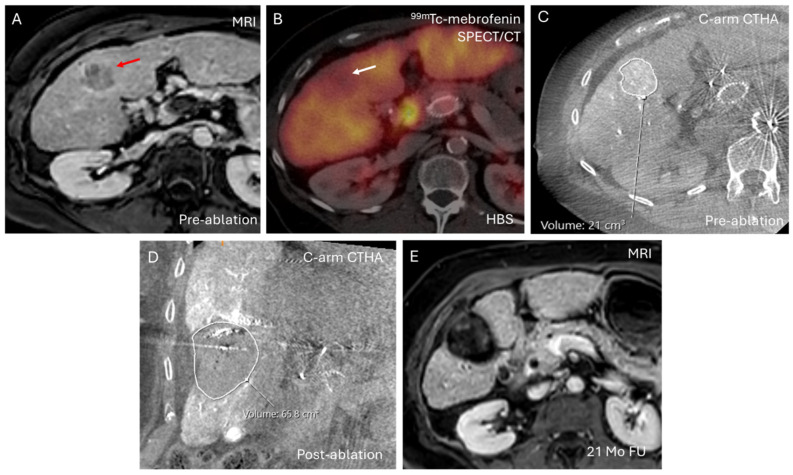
Case example of a patient with severely impaired liver function (1.6%/min/m^2^) who underwent ablation of a large HCC (maximum diameter: 40 mm). (A) Pre-treatment MRI (T1-weighted + gadolinium) showing the target lesion (red arrow); (B) ^99m^Tc-mebrofenin SPECT/CT from hepatobiliary scintigraphy (HBS) demonstrating a photopenic area (“cold spot”) corresponding to the tumor (white arrow); (C) Intraprocedural C-arm CT hepatic arteriography (C-arm CTHA) depicting the target HCC (volume = 21.0 cm^3^); (D) Post-ablation C-arm CTHA (coronal) showing the ablation zone (volume = 65.8 cm^3^); (E) Follow-up MRI (T1-weighted + gadolinium) at 21 months demonstrating no tumor recurrence or progression. Despite the severely impaired baseline liver function and the relatively large ablation volume, the patient showed no signs of post-ablation liver failure.
